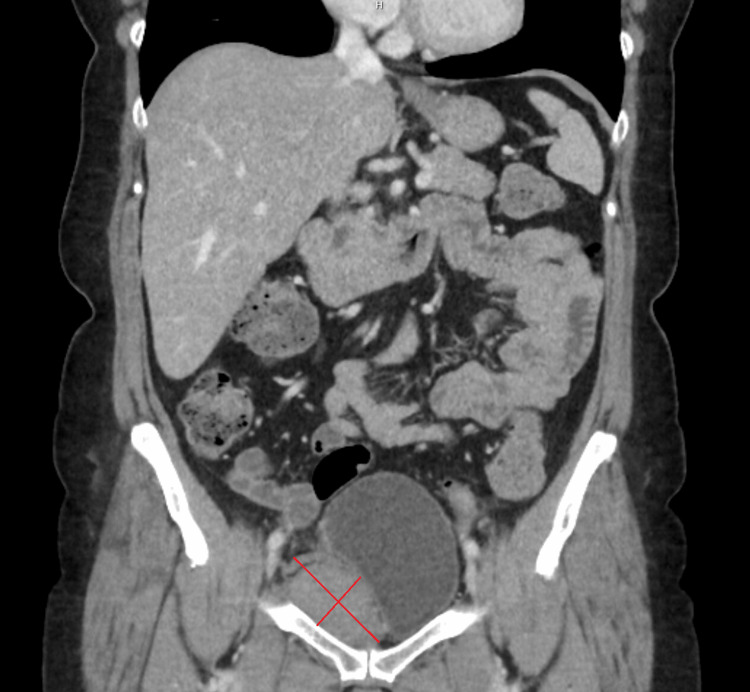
A computed tomography performed four days after intradetrusor botulinum toxin A injections revealed a large pelvic hematoma dislocating the bladder. Urethral dislocation, in combination with the intradetrusor injections, resulted in the need for intermittent catheterization.
